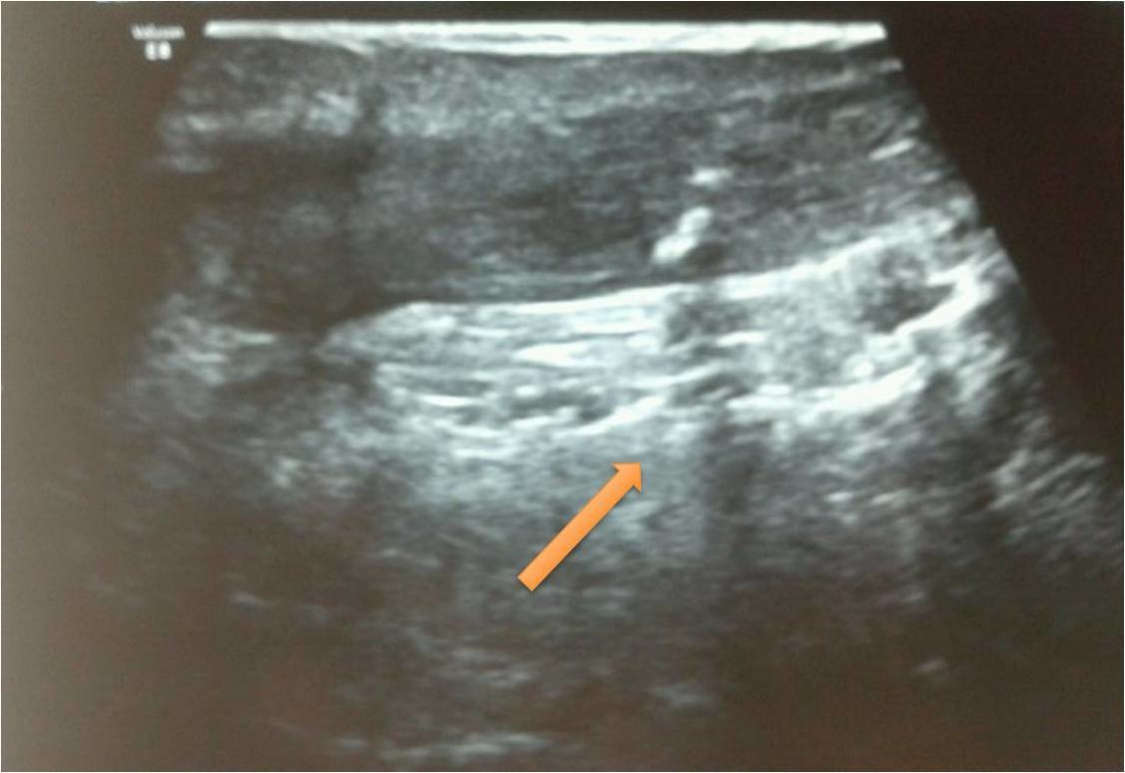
Ultrasonography of left Achilles tendon showing partial rupture
